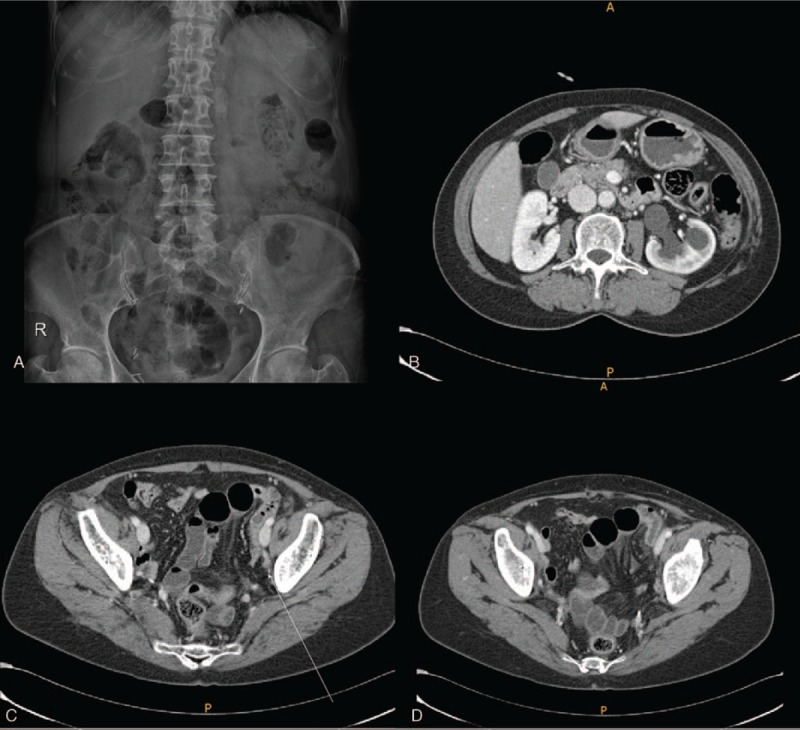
Plain radiography and computed tomography findings. A. Plain radiographs showing a non-specific bowel gas pattern. B. Left-sided hydronephrosis is observed. C. Arrow indicates abrupt luminal narrowing of the left distal ureter secondary to a suspected left distal ureteral stricture. D. CT scan showing segmental luminal dilatation of the pelvic ileal loops and 2 transition zones with the beak sign in the left-sided pelvic cavity. Reduced enhancement of the bowel loops is visualized. Mesenteric congestion is observed, and fluid collection is visualized in the pelvic ileal loops. Findings are suggestive of suspected closed-loop obstruction of pelvic ileal loops with strangulation secondary to an adhesive band. The CT scan additionally shows a post-hysterectomy state.
